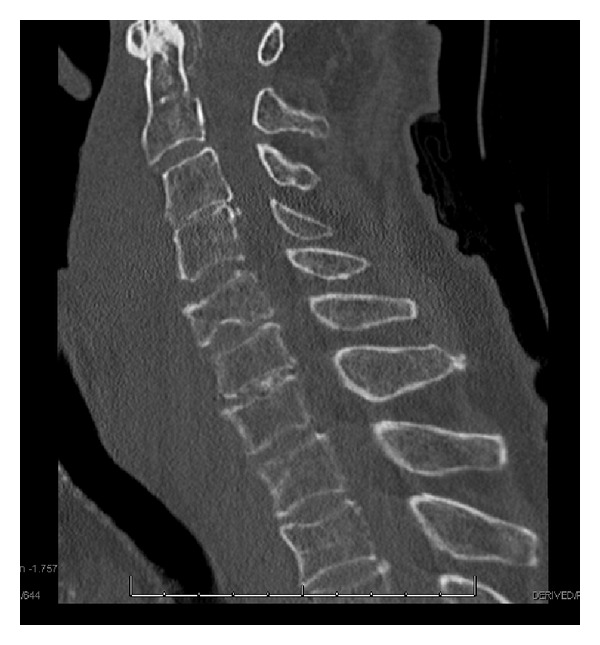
CT soft tissue of the neck without contrast showing prevertebral soft tissue swelling.
